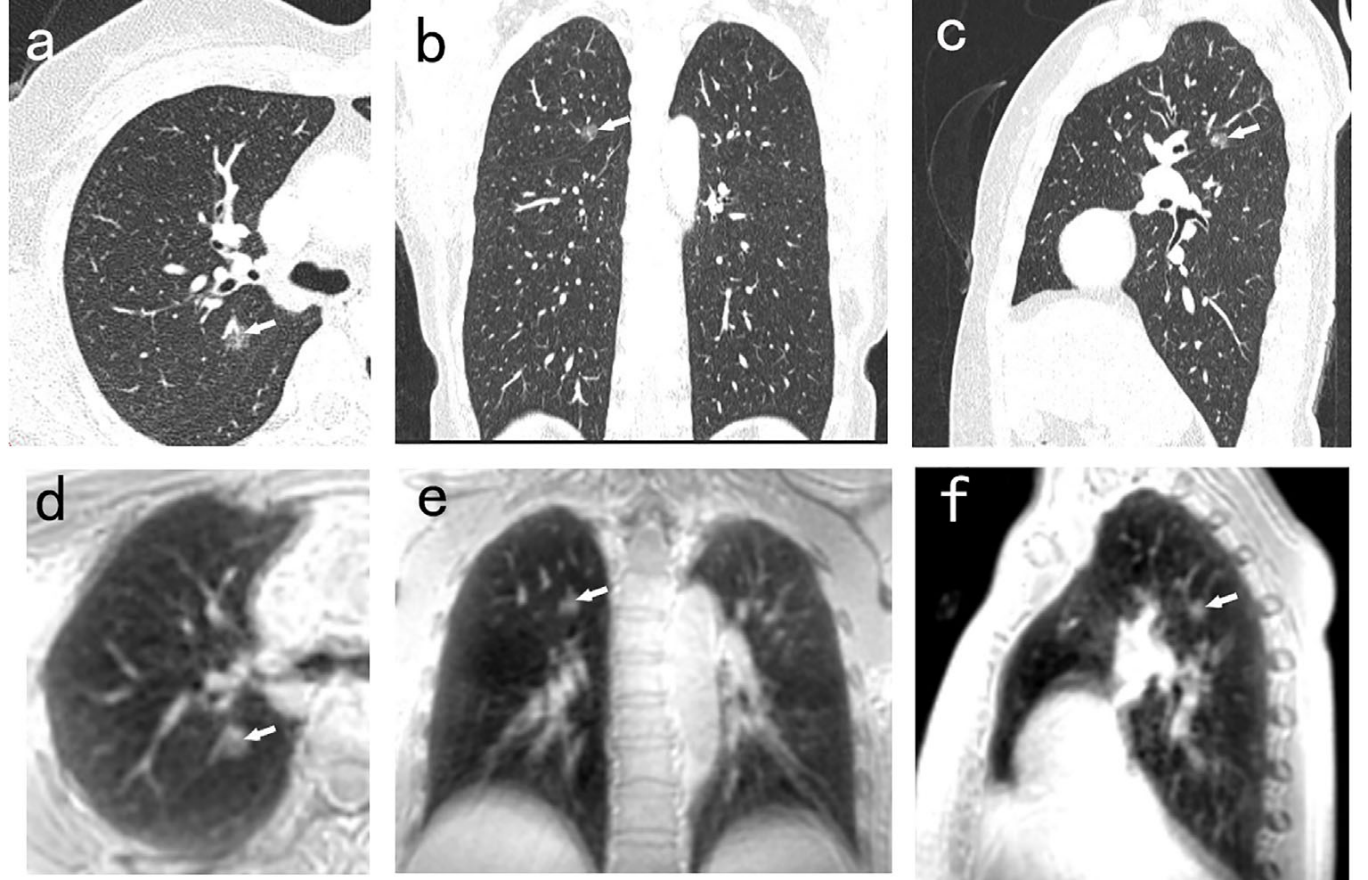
A 50-year-old man with 8.8mm GGN (arrow) in the right upper lobe. The nodule is well depicted on the CT axial (A), coronal (B), and sagittal (C) image, and on the PETRA sequence axial (D), coronal (E), and sagittal (F) image. The arrows represent the locations of nodules.
